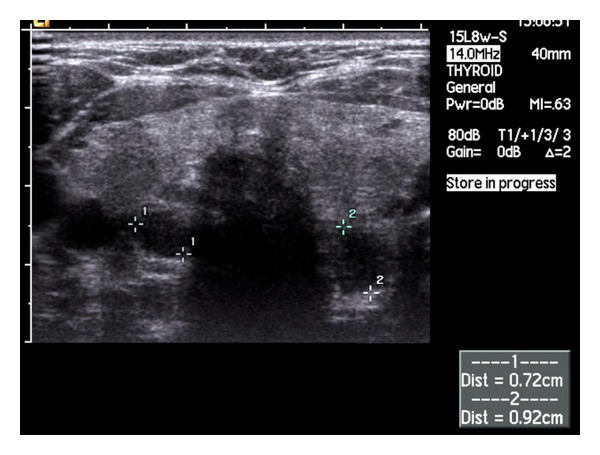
An ultrasound scan demonstrating hypoechoic nodules (outlined by caliper markings) inferior to both thyroid nodules highly suggestive of parathyroid adenomas.
